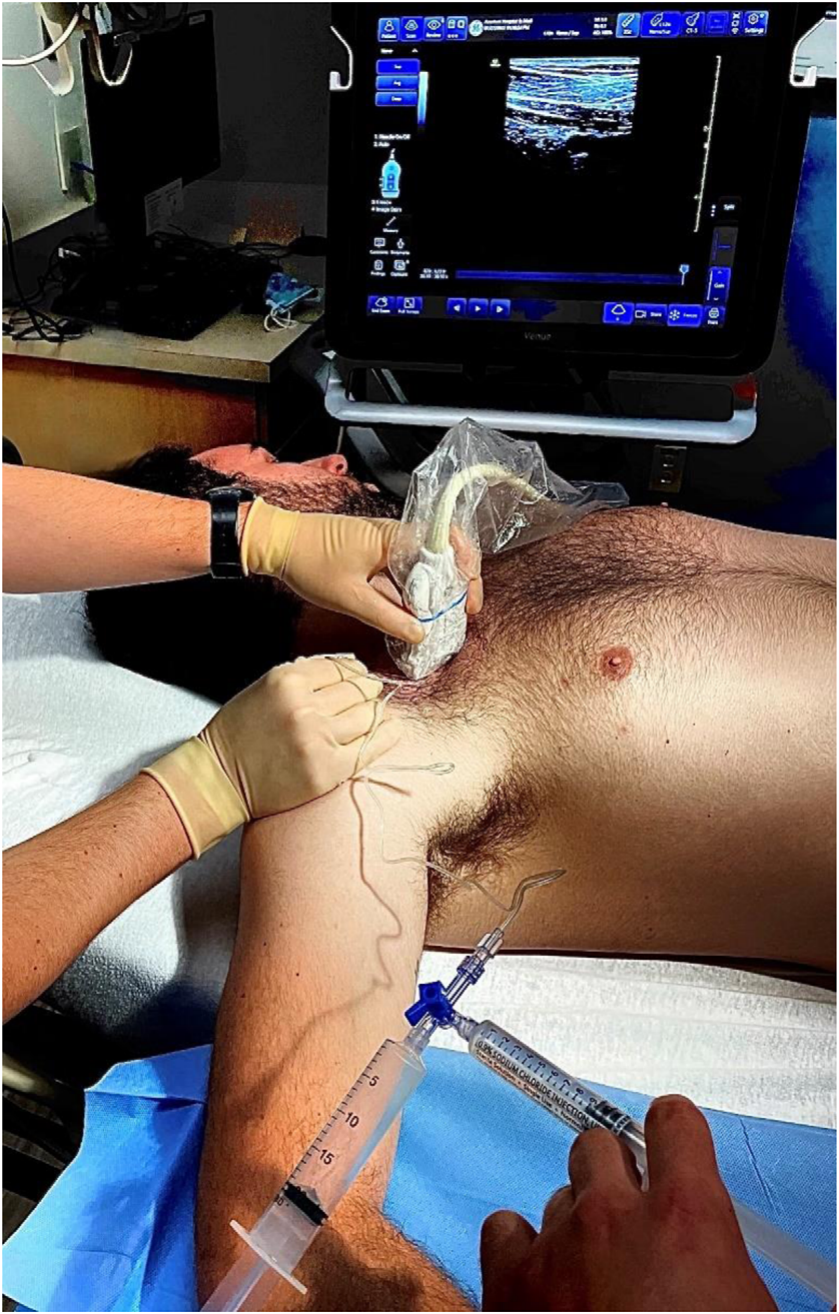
Right-sided costoclavicular brachial plexus block performance. The patient is positioned supine with ipsilateral arm abducted 90 degrees. The high-frequency linear array probe is positioned transversely just below the midpoint of the clavicle in the infraclavicular fossa. The probe beam is angled slightly cephalad to visualize the costoclavicular space posterior to the clavicle. The block needle is inserted lateral-to-medial using a standard in-plane technique. A two-person block technique is used, whereby one clinician performs dynamic needle guidance under ultrasound while the other administers local anesthetic.
